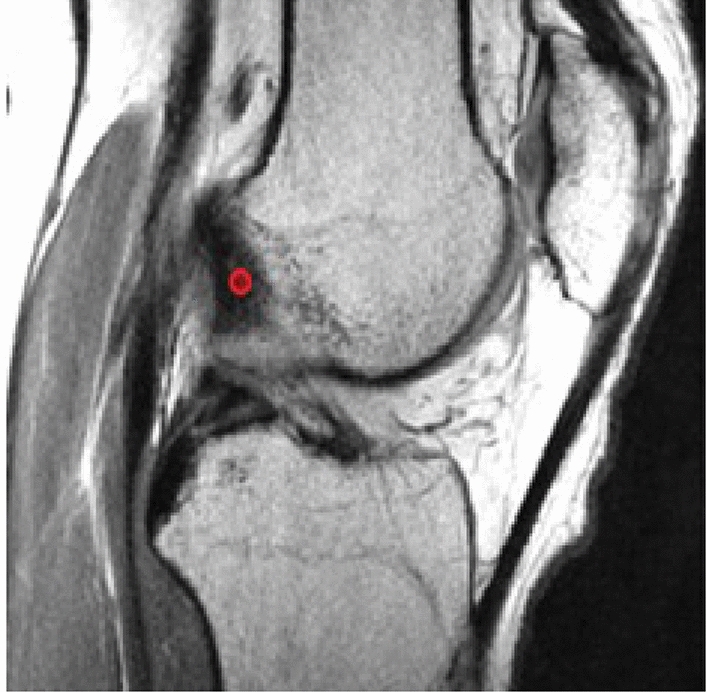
Example of sagittal view of a 3D MRI. The center of the femoral origin of the ACL was determined and marked by a red circle of 2 mm in diameter
